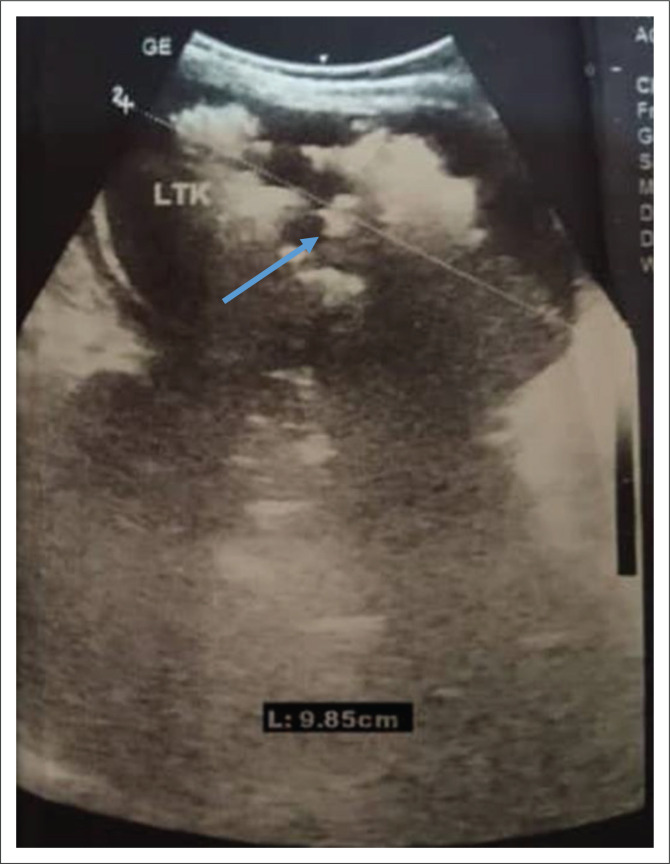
Sonogram of the left kidney of an emphysematous pyelonephritis patient at the Usmanu Danfodiyo University Teaching Hospital, Sokoto, Nigeria, November 2019. Figure shows renomegaly, reduced renal parenchyma echogenicity and multiple irregular mixed echoes with dirty shadowing (blue arrow).
